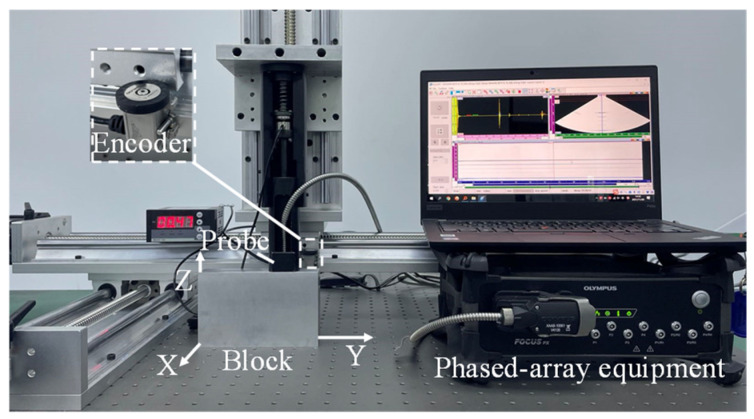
The ultrasonic testing setup.
